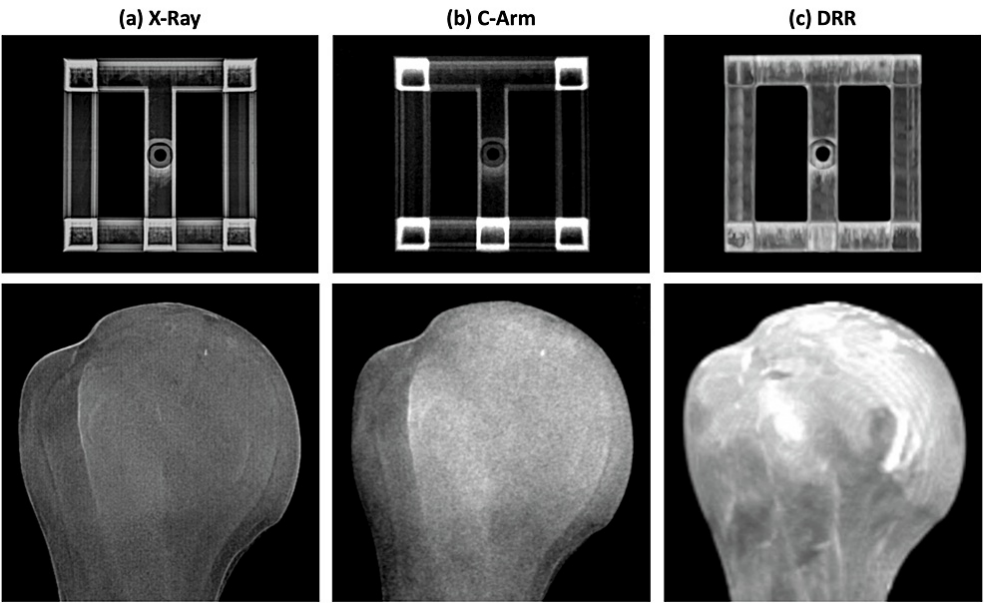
Treated images of the 3D printed prototype (upper raw) and the anatomical humerus bone model (lower row) used for analysis. (a) X-ray image, (b) C-arm fluoroscopic image, (c) digitally reconstructed radiography (DRR). The total surface area was calculated in cm^2^.
